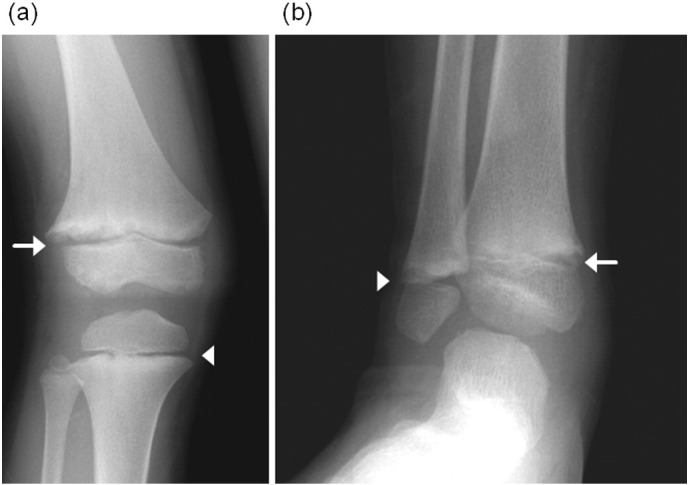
X-ray pictures of the knee (a) and the ankle (b) of the patient at the age of seven. (a) Metaphyseal fraying and flaring of the distal end of the femur (arrow) and metaphyseal fraying of proximal end of the tibia (arrowhead). (b) Metaphyseal fraying and flaring in the distal end of the tibia (arrow) and the fibula (arrowhead).
